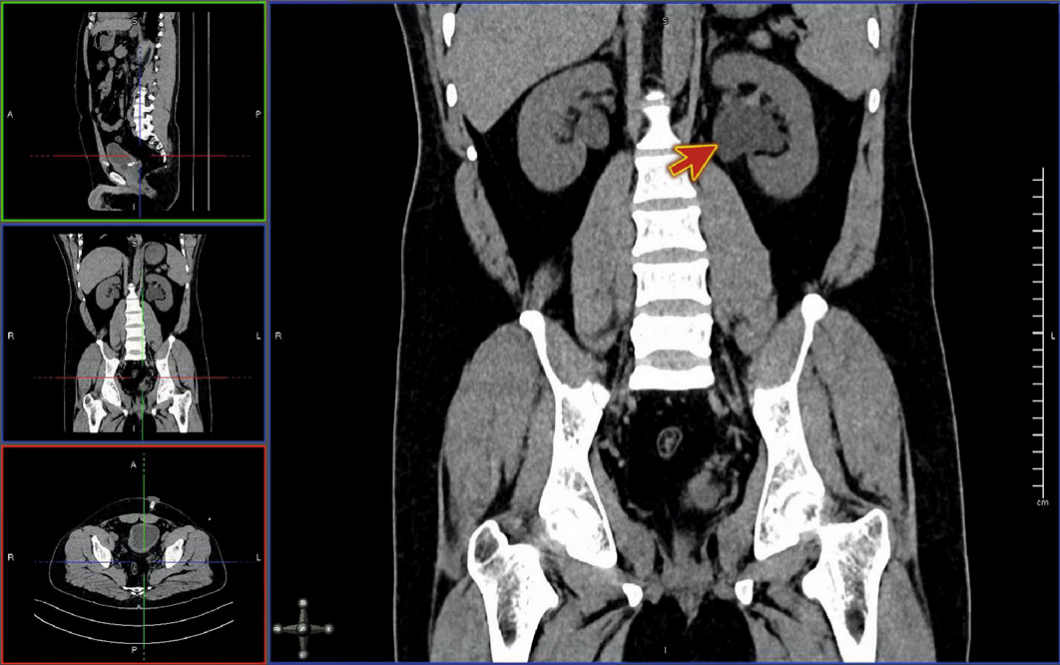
Computed tomography (CT) abdomen, coronal view showing moderate dilatation of the left pelvicalyceal system (red arrow).
